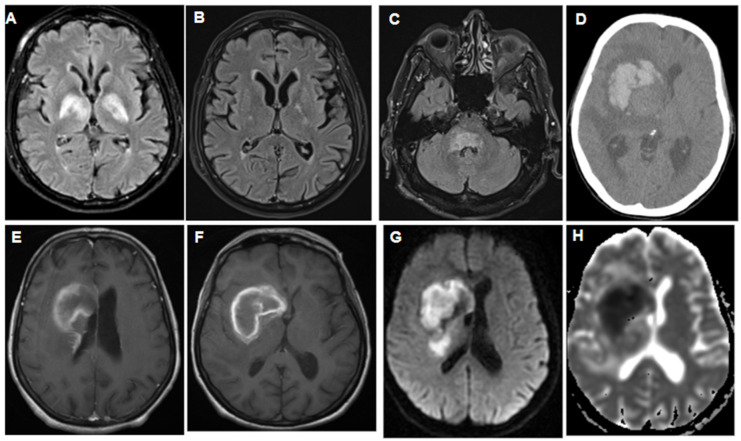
A series of imaging findings of patients with PCNSL and various neurological symptoms, demonstrating initial presentation, treatment response, and follow-up imaging, providing insights into the diagnostic process and radiographic treatment outcomes. (A): T2-flair MRI with gadolinium of a 79-year-old patient presenting with ataxia and episodes of delirium, showing bi-thalamic hyperintensity. (B): T2-flair MRI with gadolinium of the same patient after treatment, demonstrating evidence of near-complete resolution. (C): T2-flair MRI with gadolinium of a 64-year-old patient presenting with 6 months of ataxia and dizziness. (D): A CT scan of a 64-year-old patient with left hemi-syndrome, initially presenting as a hemorrhage. (E,F): T1+gadolinium MRI scan of the same patient exhibiting the same hemorrhage with contrast enhancement, as well as small periventricular gadolinium enhancement that was not visible in the earlier CT scan. (G): Diffusion-weighted imaging (DWI) of the hemorrhagic lesion, confirming the diagnosis of hemorrhagic stroke. (H): ADC (apparent diffusion coefficient) MRI series of the same patient, providing additional information about the hemorrhagic stroke.
